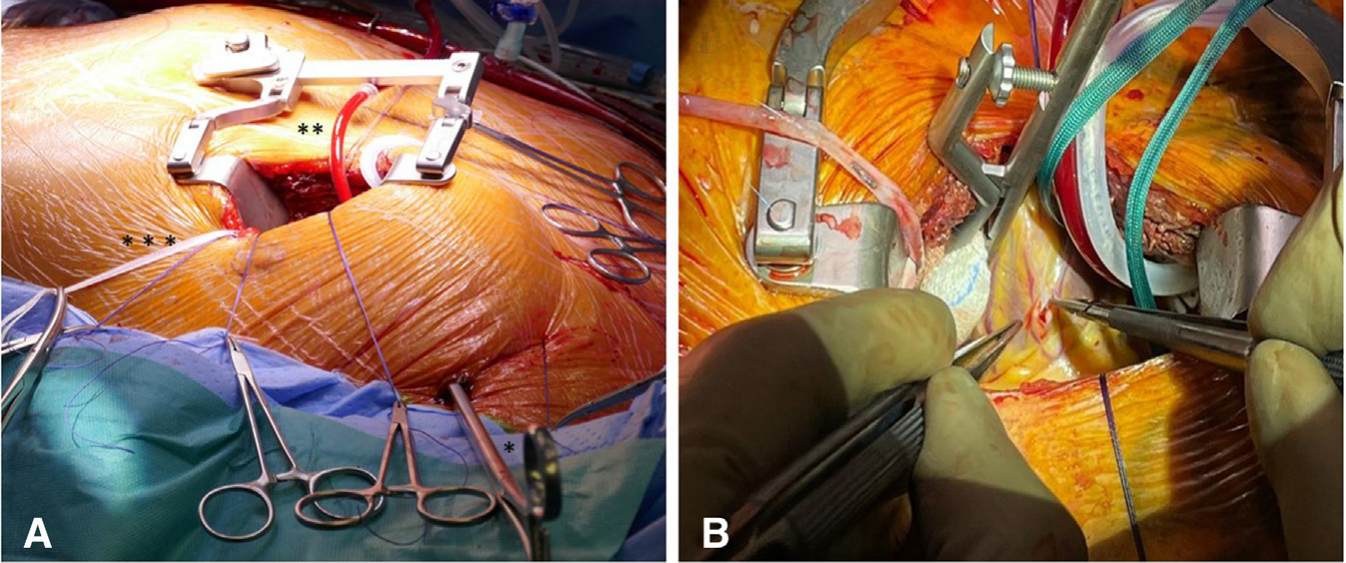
Left anterior minithoracotomy (fourth intercostal space) preparation and incision of coronary arteries. ∗Transthoracic cross-clamp, ∗∗cardioplegia line, ∗∗∗aortic sling.
